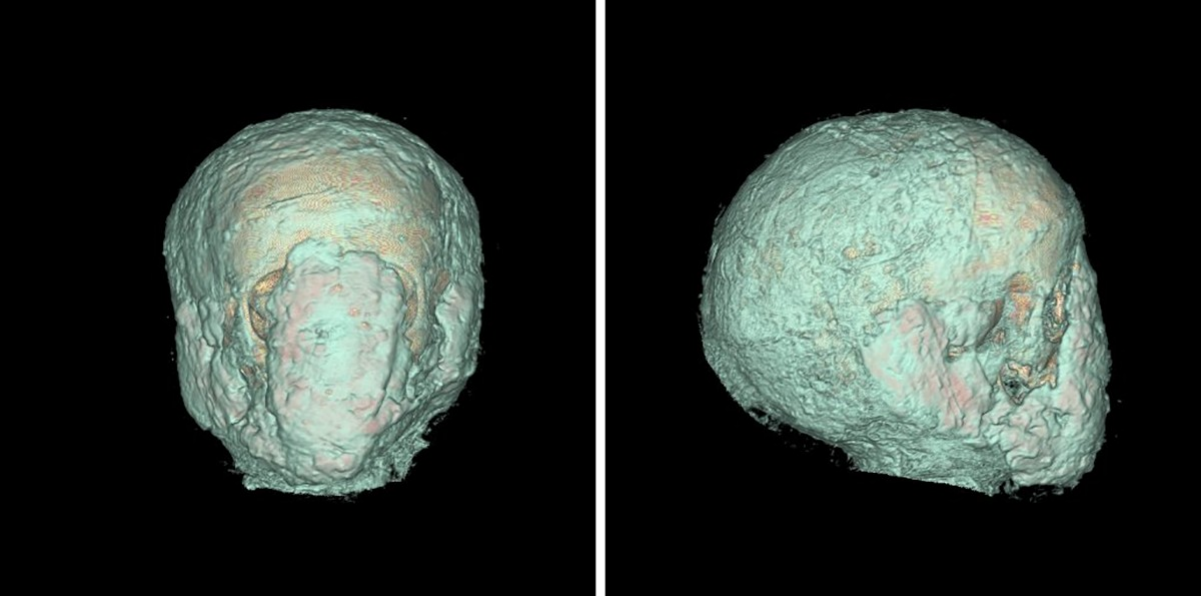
Three-dimensional reconstruction of the CT scans. This shows an amorphous material mass over the face–obviously for the proper fixation of the portrait; left side: frontal view; right side: lateral view.
